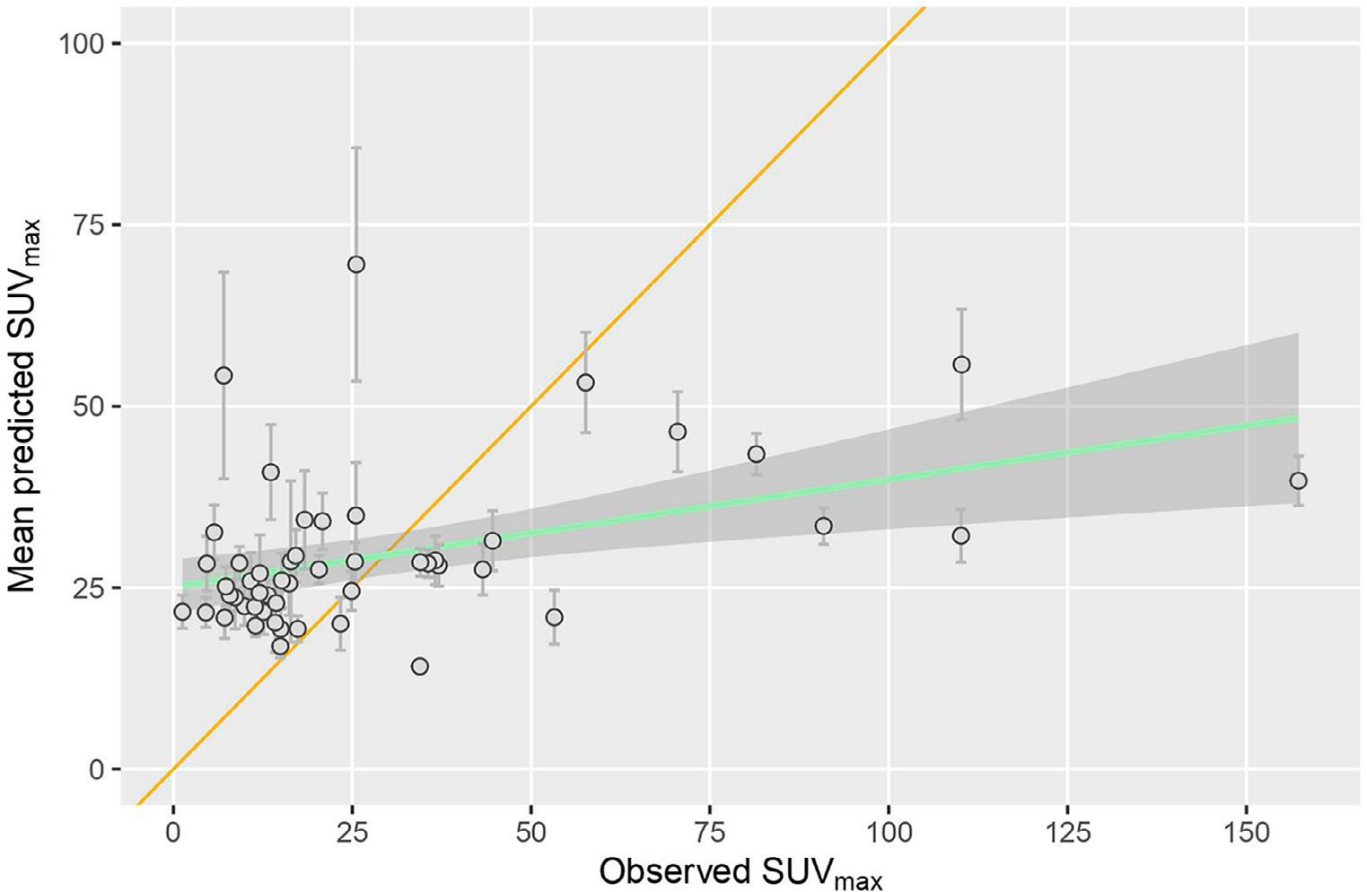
Mean SUV_max_ values predicted by the random forest models plotted against the observed SUV_max_ values for the 51 considered patients. The error bars indicate the standard deviation. The yellow line is the identity line. The green line and corresponding shaded area represents a linear regression fit to the data and its confidence interval
